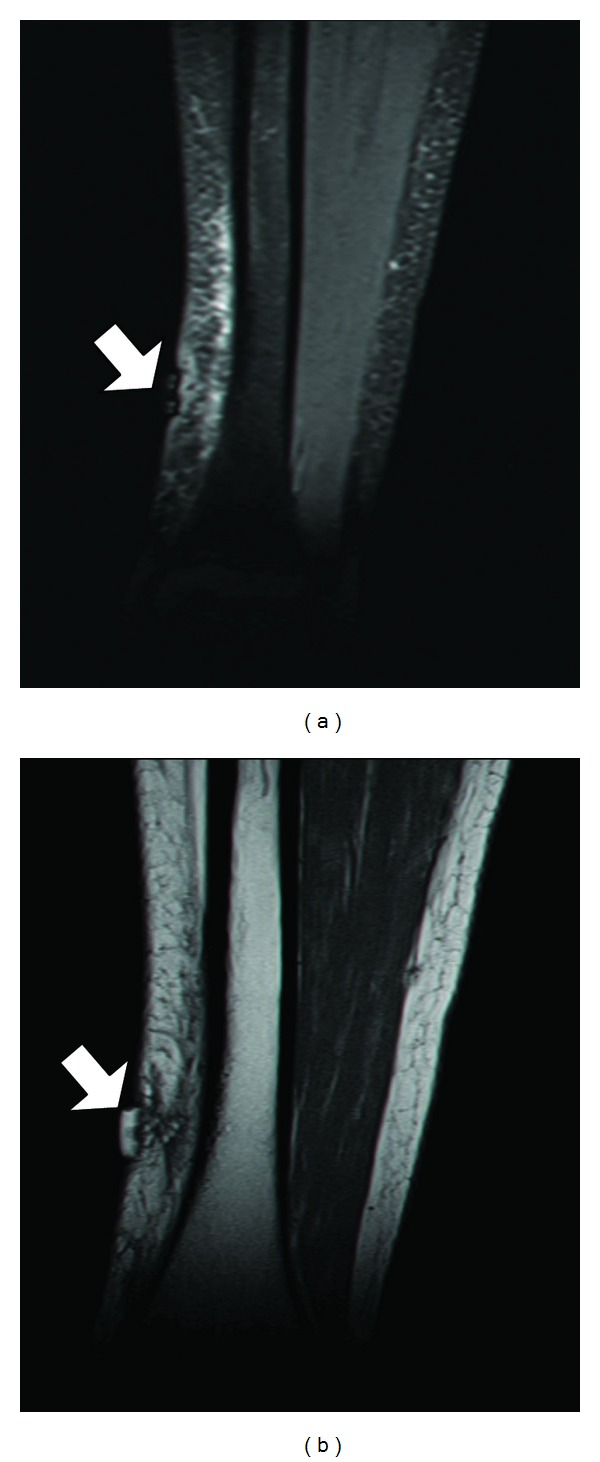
(a) MRI-T1 tirm and (b) MRI-T1. Coronal slices of the distal part of shin with cutaneous tag. MRI findings were edema in subcutaneous fat medial to tibial bone with thickening of subcutaneous septations and unclear boundaries, without involvement of bone cortex or marrow. T2-weighed images did not add information and are not shown.
